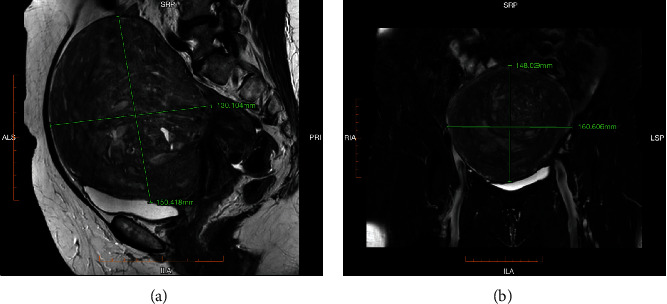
MRI of the pelvis. (a, b) Large leiomyoma arising anteriorly from the body and cervix of the uterus, measuring 150 × 130 × 150 mm, with central cystic and necrotic changes and displacement of the uterus left posteriorly. Multiple smaller fibroids up to 25 × 20 mm and mild distension and dilatation of both ureters also seen.
